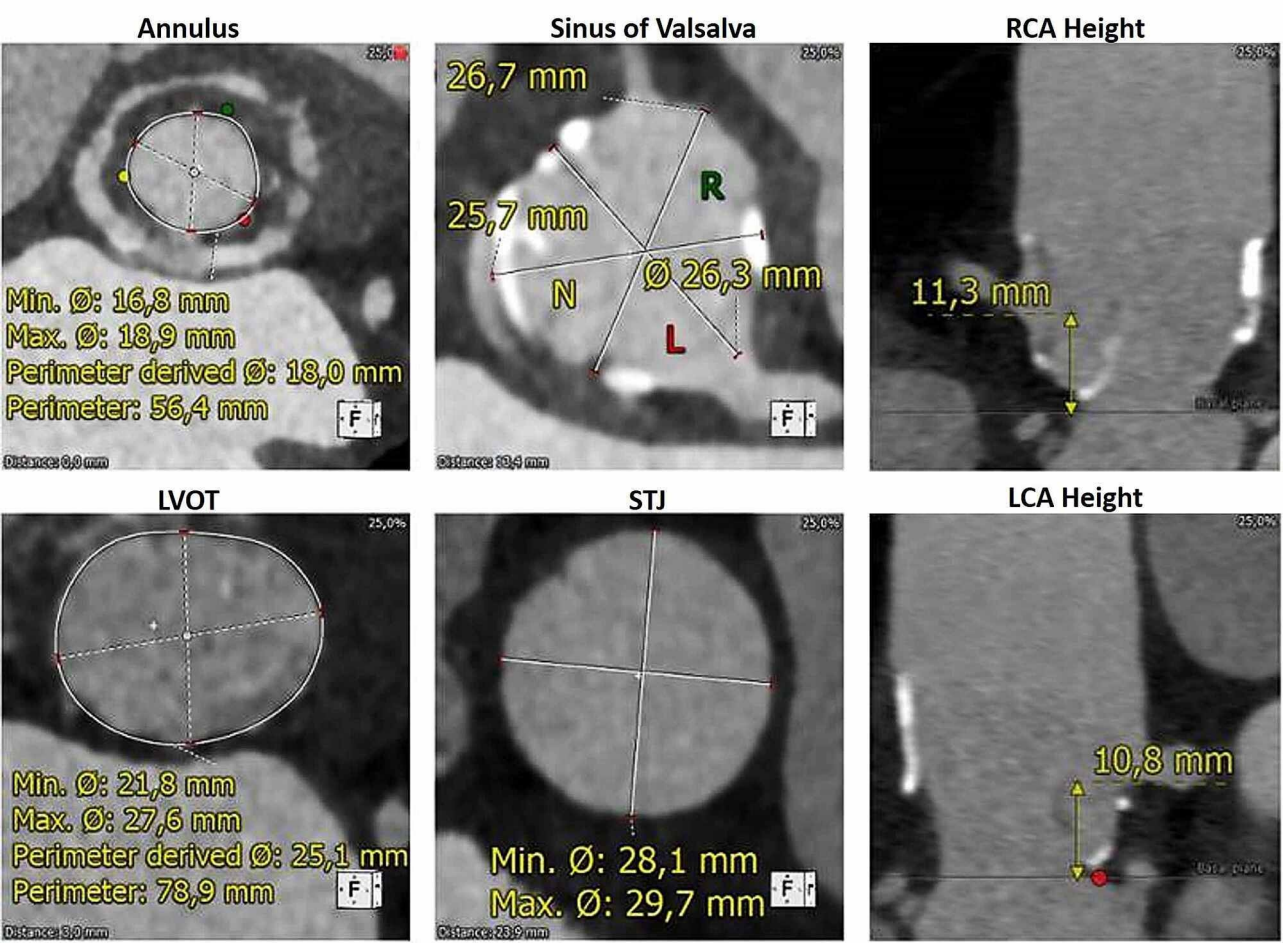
Pre-procedure CT scan LCA - left coronary artery; LVOT - left ventricle outflow tract; RCA - right coronary artery; STJ - sino-tubular junction
